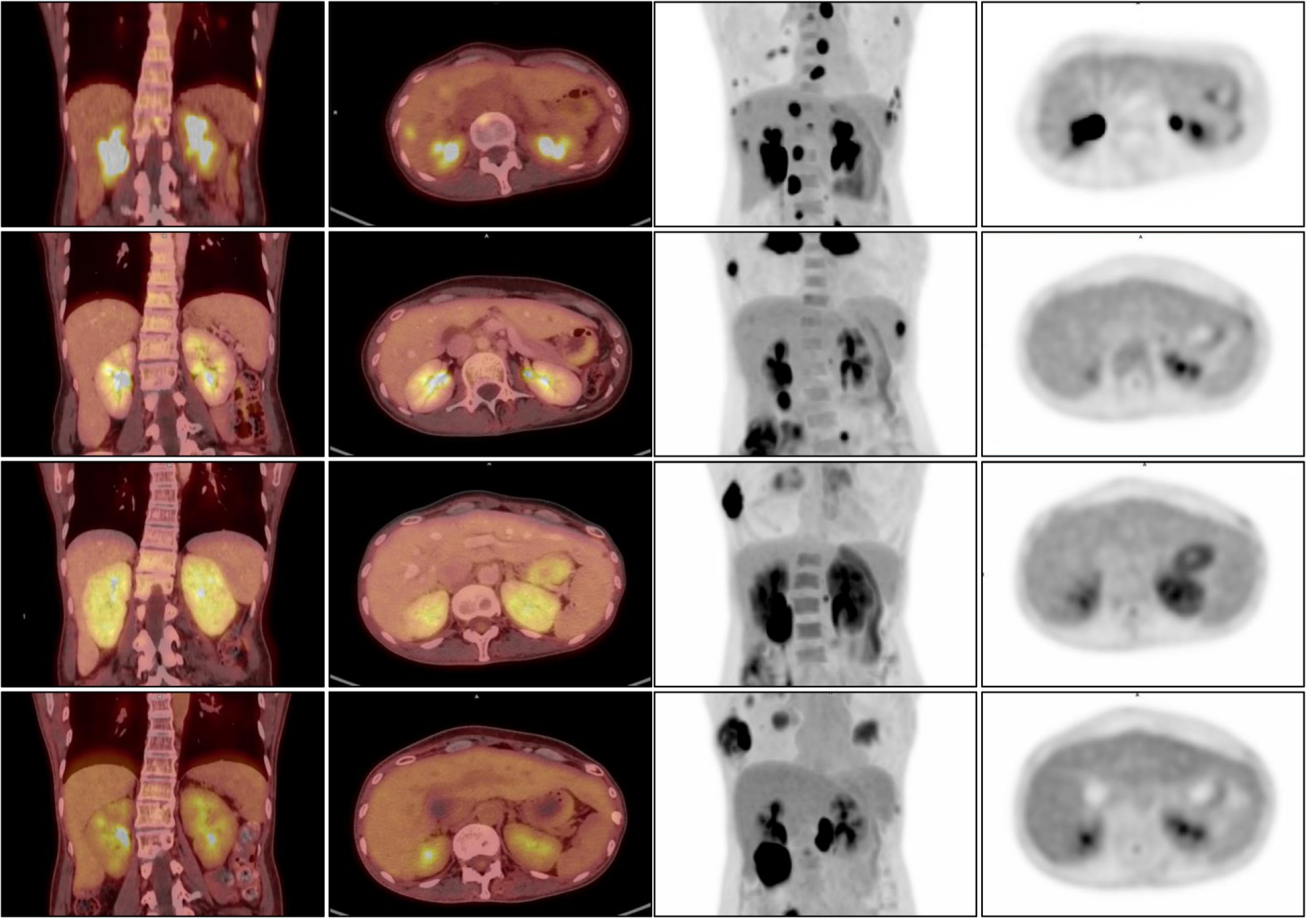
PET-CT scans obtained before and during patient’s treatment course. The initial scan (a) was obtained prior to immunotherapy treatment, and the subsequent scan (b) was performed after initiation of immunotherapy. Both (b) and (b) were performed at baseline renal function, and results of the scans show normal renal parenchymal FDG uptake and expected excretion of FDG into the renal pelvises. The third scan (c) was obtained during the patient’s AKI, and demonstrates markedly increased FDG uptake within the renal parenchyma compared to (a) and (b). Following steroid treatment and recovery of renal function, a fourth scan (d) was obtained, showing a return to baseline renal parenchymal FDG uptake, consistent with resolution of interstitial inflammation. Abbreviations: *PET-CT* positron emission tomography–computed tomography, *FDG* fluorodeoxyglucose
